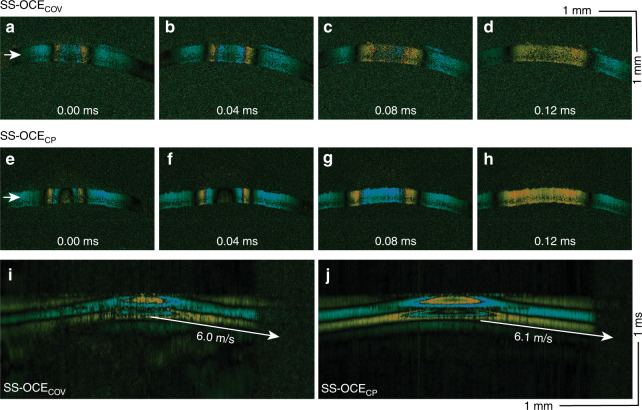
Elastic wave in rabbit cornea. a–d Time-lapse Doppler OCT B-scans from the SS-OCE_COV_ system. e–h Time-lapse Doppler OCT B-scans from the common-path OCE system. i, j Spatiotemporal Doppler OCT at a depth indicated by the white arrows in (a) and (e), respectively
